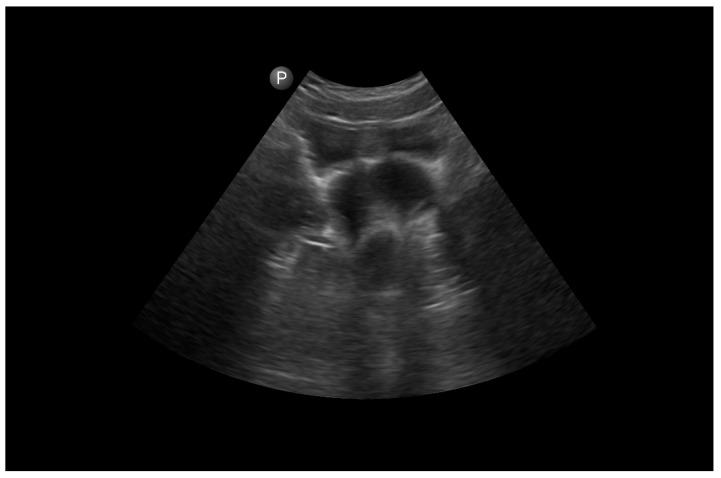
Intraoperative abdominal sonography and drainage of hematocolpos. P is polish equivalent to R-right in english site of the ultrasound screen.
